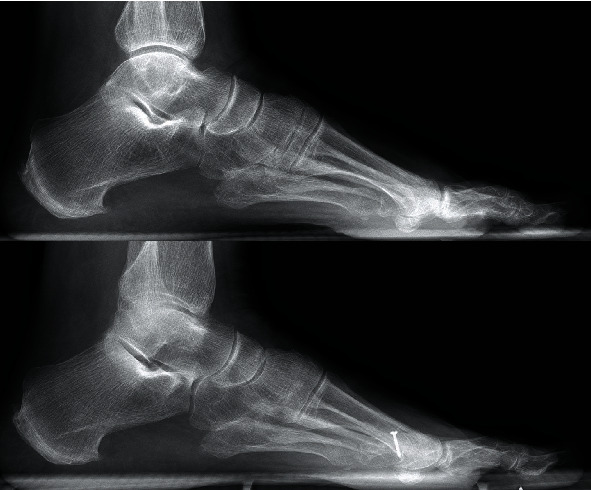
Lateral radiograph prior surgery and at three months follow-up.
